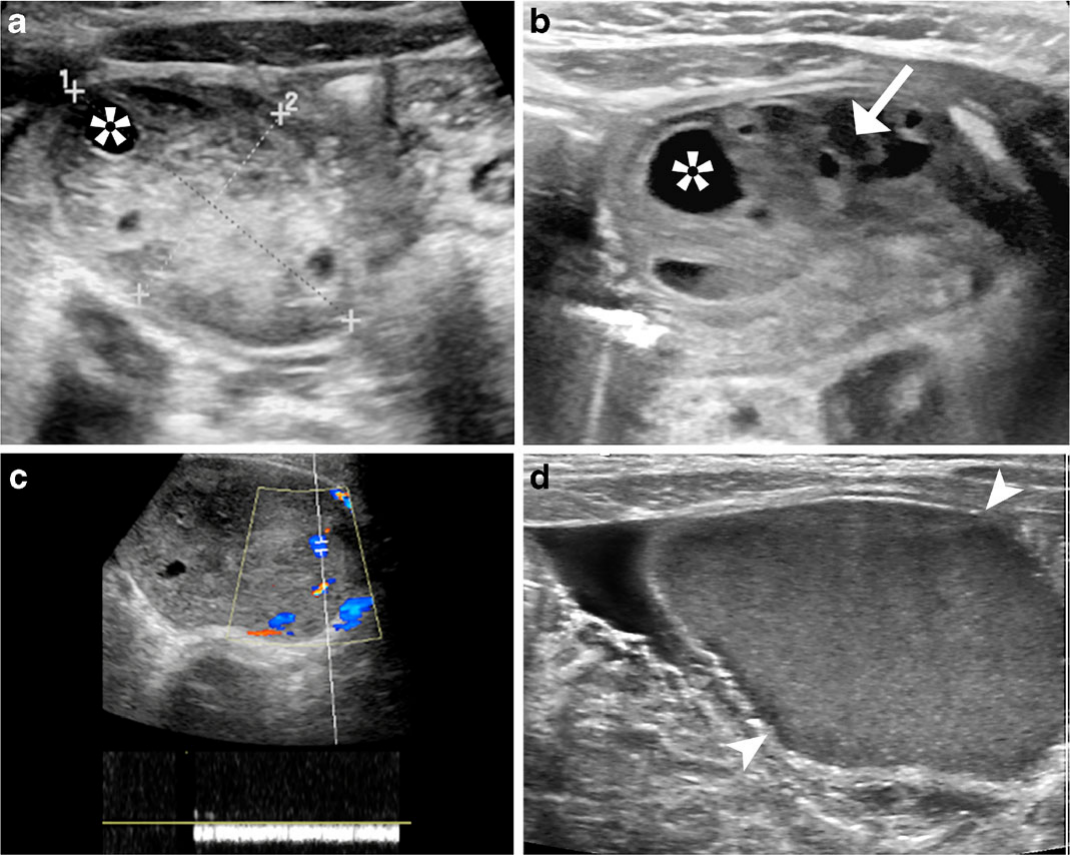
Typical US findings in childhood ovarian torsion. a The initial US of the lower abdomen in a pre-pubertal girl shows an enlarged ovary (*calipers*) with peripheral follicles (*asterisk*), consistent with an ovarian torsion. b Follow-up some days later in the same girl as in (a) shows cystic ovarian compartments (asterix) with sedimented echoes (arrow) indicating progressive hemorrhagic infarction. c US of the lower abdomen with a linear transducer performed for lower quadrant pain in a pre-pubertal girl depicts an enlarged, partially cystic ovary with stromal vessels on color Doppler (venous flow pattern) indicating that this probably is an ovarian tumor (with or without partial torsion). d US of the lower abdomen in a newborn girl. Axial section with a linear transducer shows an ovarian cyst (*arrowheads*) with internal echoes probably after hemorrhage. Even if large, this complicated neonatal ovarian cyst vanished without symptoms spontaneously within some months
